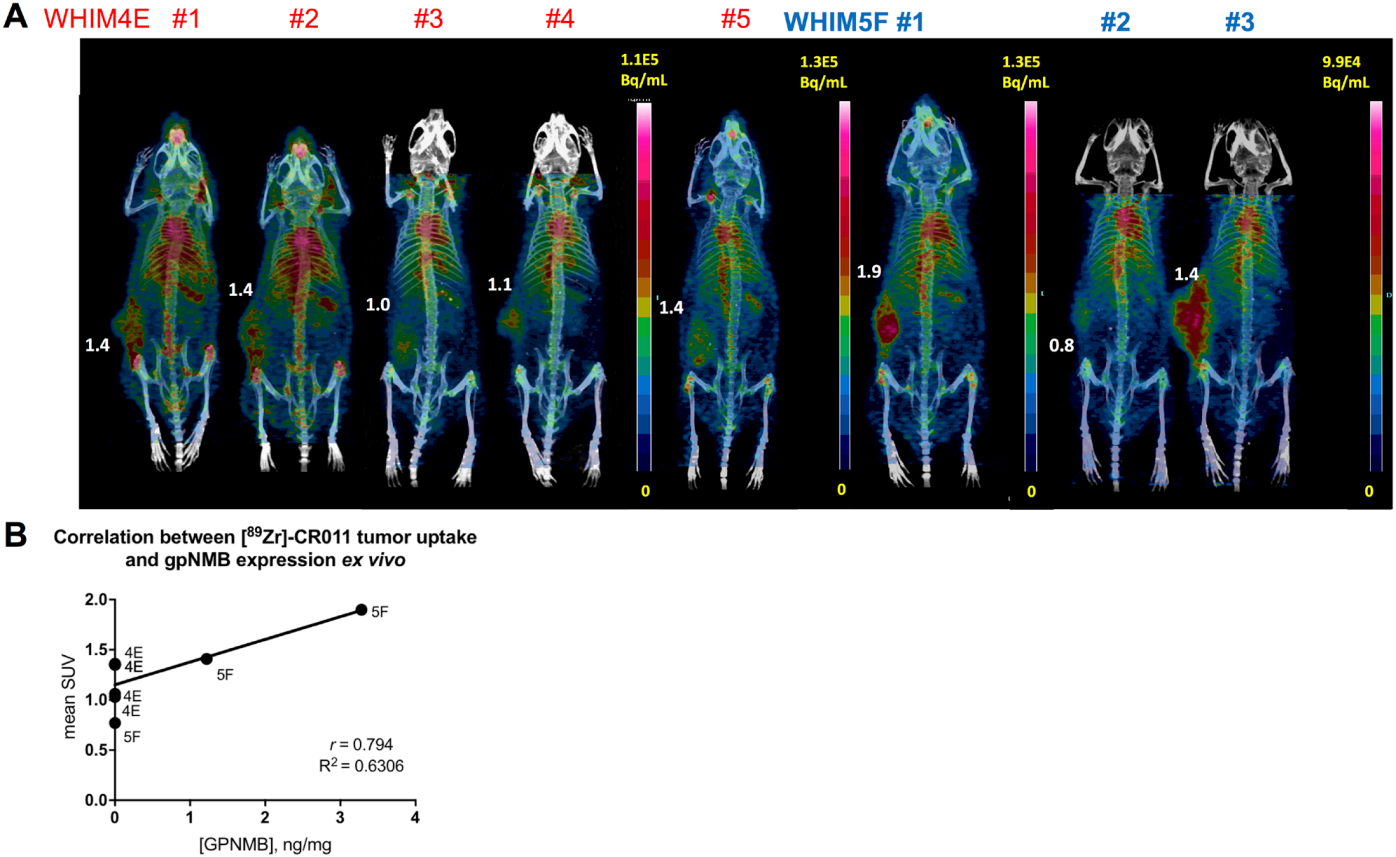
PET/CT imaging of [^89^Zr]DFO-CR011 in PDX models of TNBC at 7 days post-injection (A) Maximum Intensity Projections of WHIM-4E and WHIM-5F. Mean SUV are shown as values next to the tumors. (B) Correlative study between mean SUV in the tumor and gpNMB expression via ELISA.
